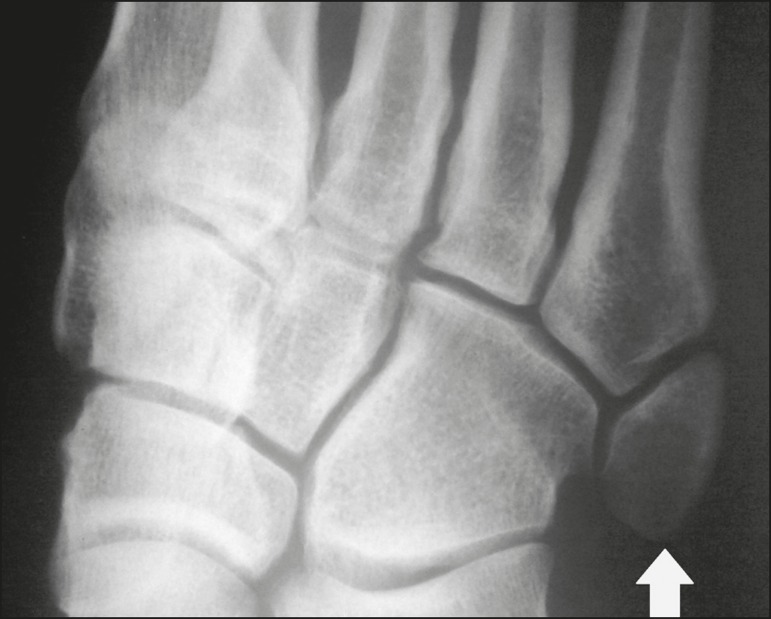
X-ray of the left foot (detail), in an oblique view, showing an unfused
